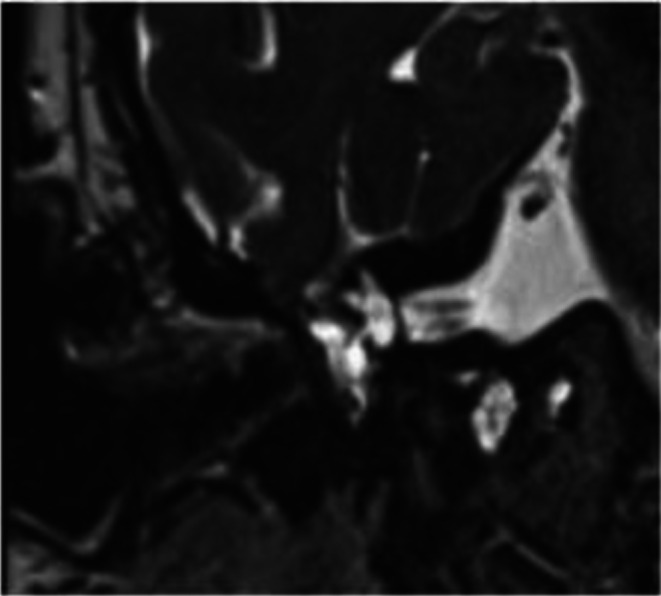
Right tegmen defect shown via coronal T2 MRI.
